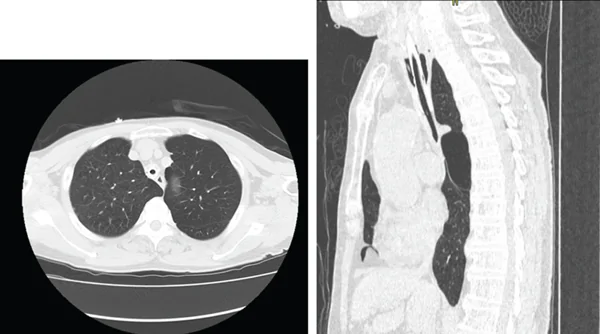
Imaging studies performed after intubation. CT scan shows narrowing of the lumen, just adjacent to the endotracheal tube.
